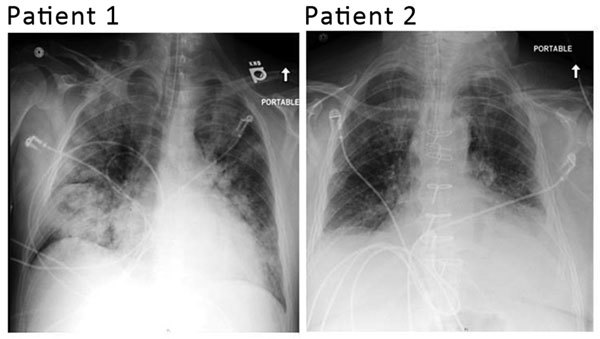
Admission chest radiographs of 2 adults with severe infections with adenovirus type 7 in family, Illinois, USA, 2014. Chest radiograph of patient 1 shows diffuse parenchymal consolidation involving all lobes. Chest radiograph of patient 2 shows interstitial changes in all lung fields and cardiomegaly.
